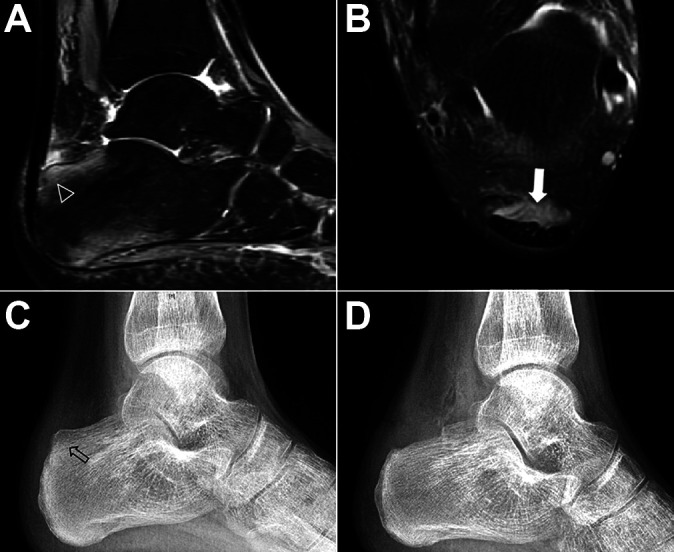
Endoscopic procedure for Haglund syndrome. (A and B) Retrocalcaneal impingement as visualized on magnetic resonance imaging. (A) Sagittal view with bone marrow edema (arrowhead). (B) Axial view with retrocalcaneal bursitis (white arrow). (C and D) Lateral ankle radiographs (C) with enlargement of posterosuperior calcaneal tuberosity with Haglund deformity (arrow) and (D) after posterosuperior calcaneal tuberosity was excised.
